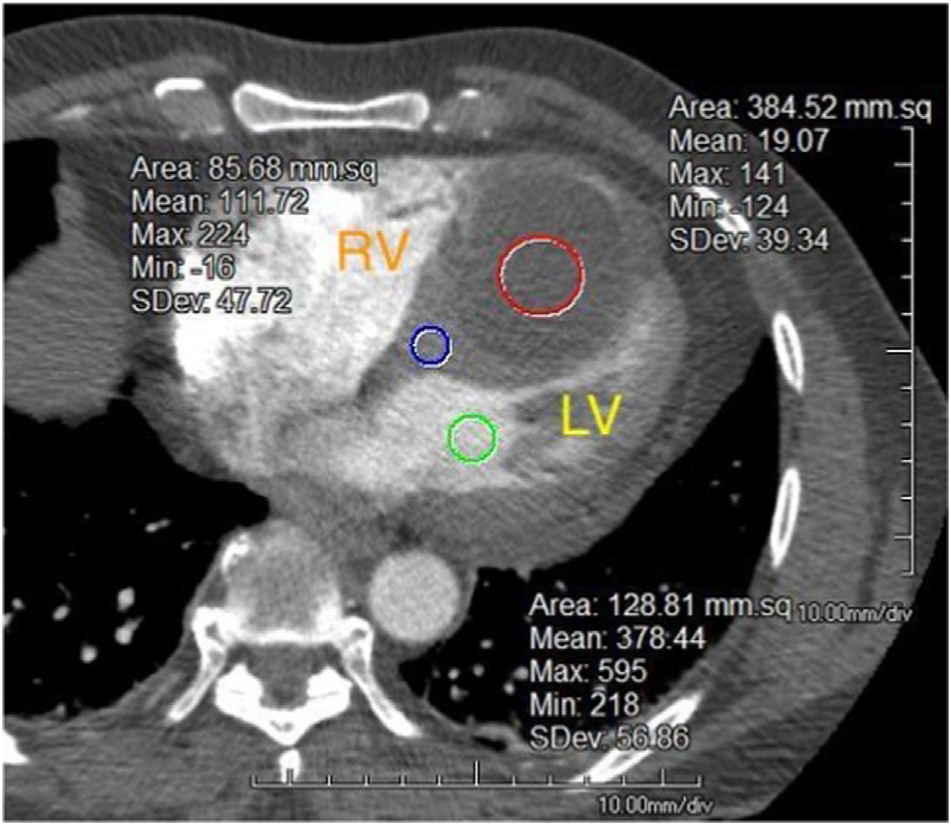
Chest CT, axial view, nongated image obtained after intravenous iodine contrast administration, demonstrating a large interventricular septal mass with low attenuation (*red circle*; mean attenuation 19.07 ± 39.34 Hounsfield units), consistent with avascular fluid content. The mean attenuation values for the IVS (*blue circle*; 111.72 Hounsfield units) and the left ventricle (*green circle*; 378.44 Hounsfield units) are also shown.
